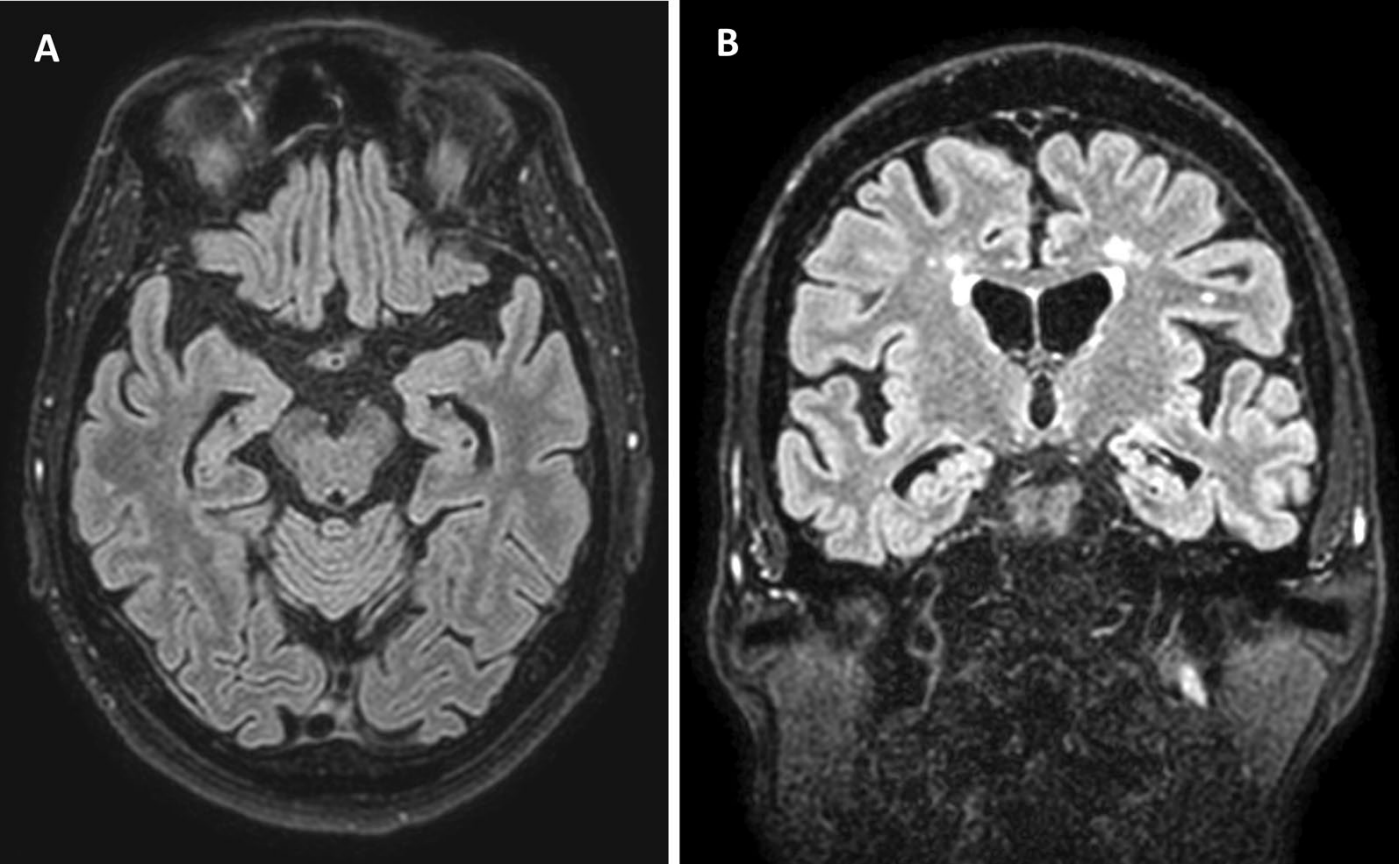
Cerebral MR imaging showing bilateral hippocampal atrophy and mild global atrophy (Fluid attenuated inversion recovery (FLAIR); A: axial; B: coronar)
